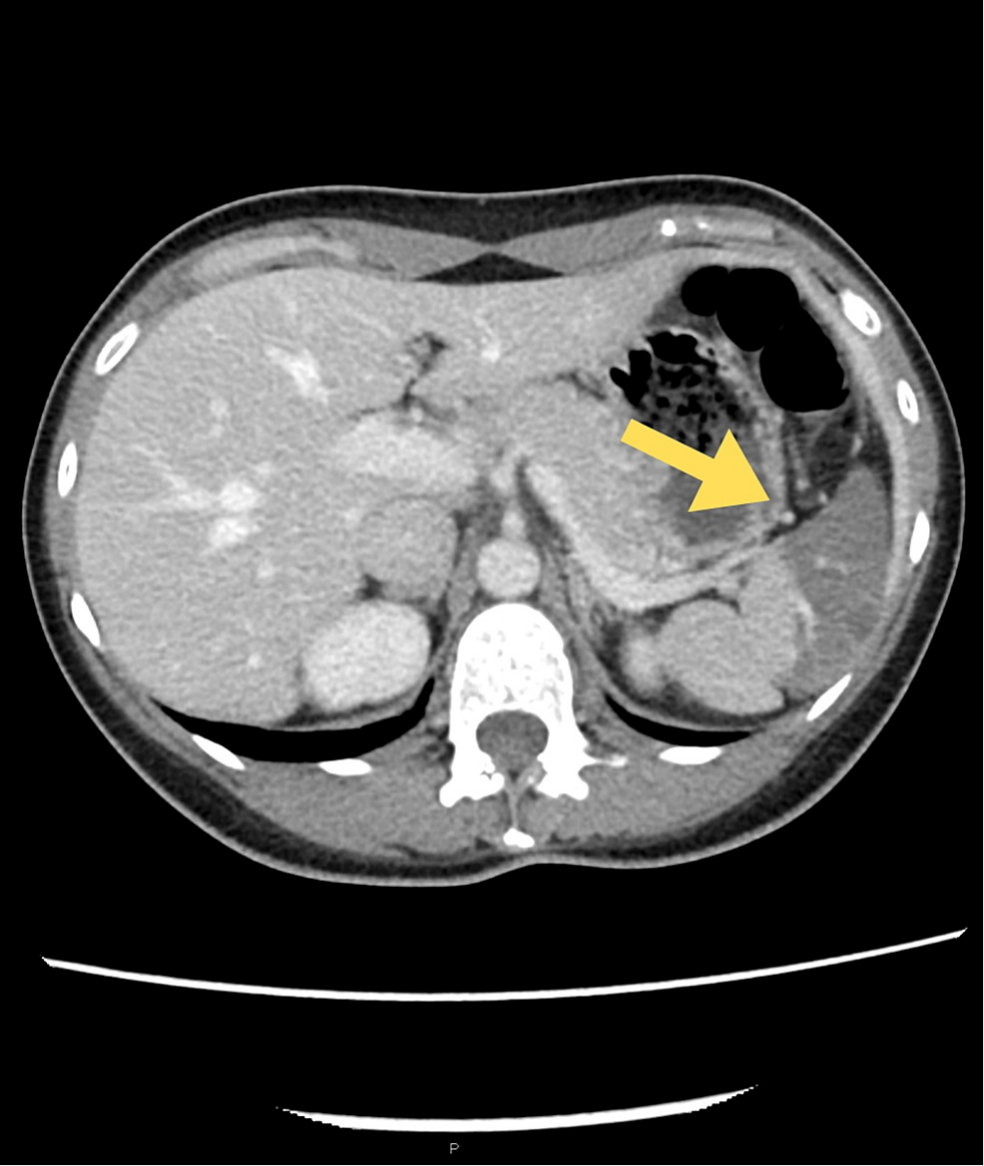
CT abdomen and pelvis with IV contrast showing a large splenic infarction
